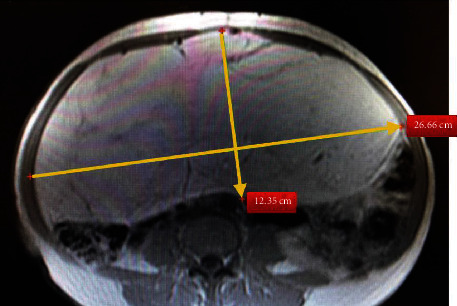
Axial view of magnetic resonance imaging shows that the lesion is encapsulated and lobulated.
